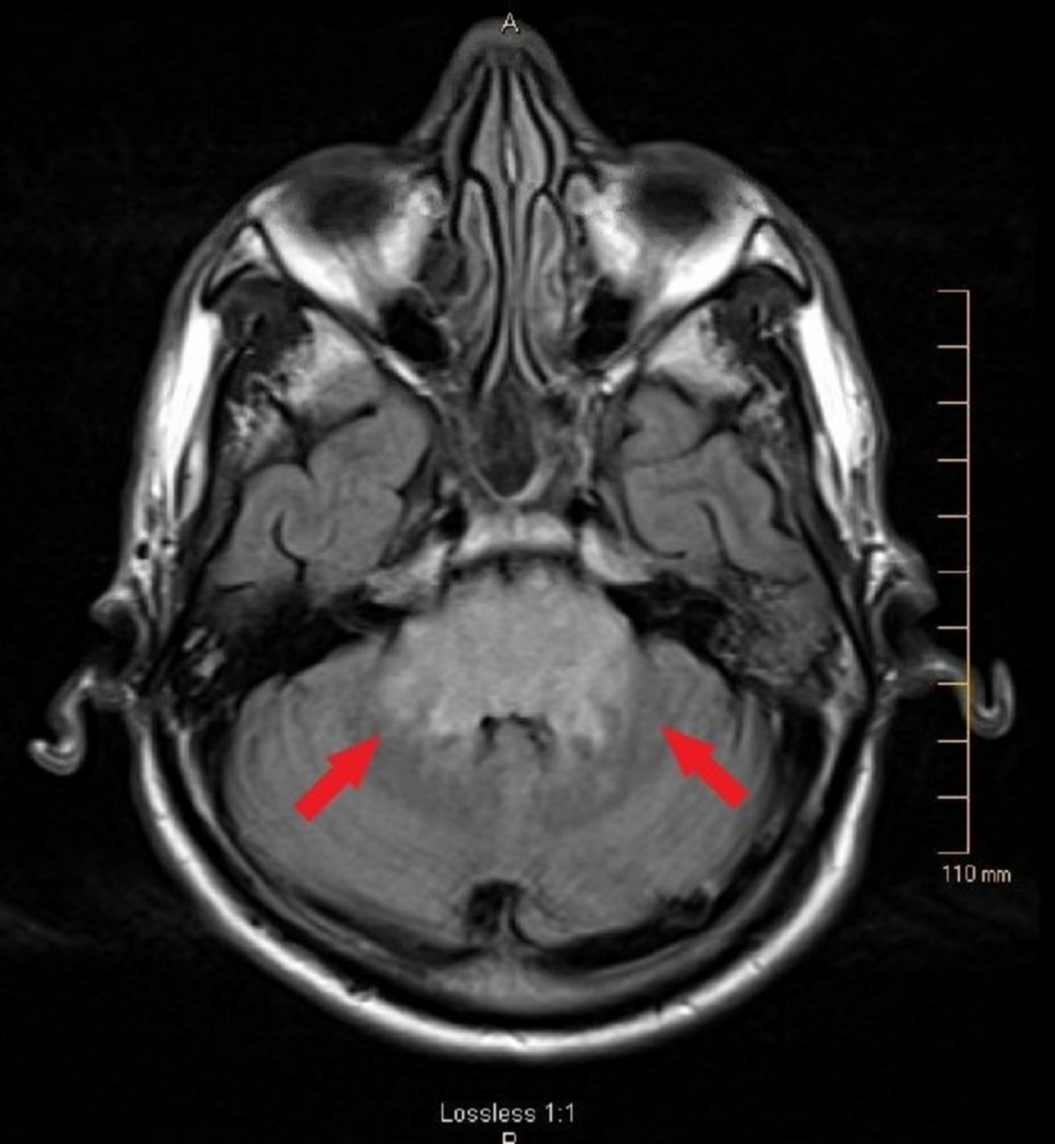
MRI on admission shows hyperintense signals on FLAIR sequence involving the brainstem and extending in the cerebellar peduncles bilaterally (red arrows). MRI: Magnetic resonance imaging; FLAIR: Fluid-attenuated inversion recovery.
